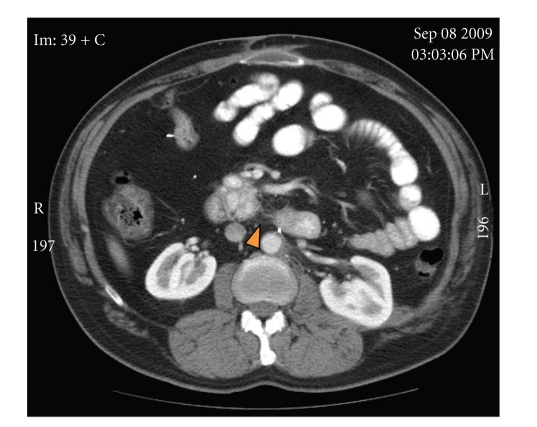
Axial contrast enhanced CT abdomen. Two years imaging follow-up shows interval decrease in size of the paraganglioma. Perilesional fat stranding is related to prior surgical procedure and radiation therapy (arrowhead).
